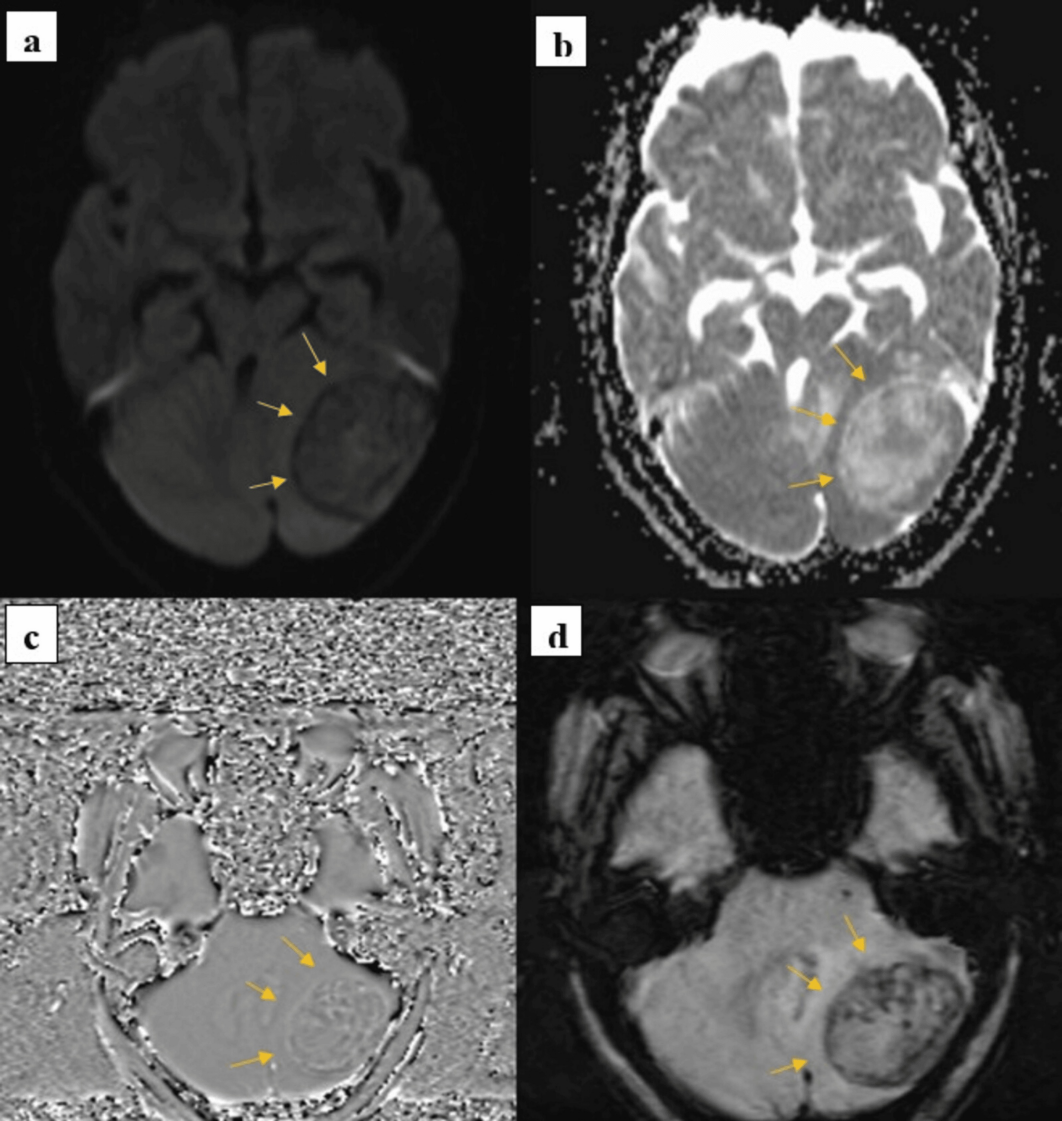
(a) Diffusion-weighted imaging (DWI) and (b) apparent diffusion coefficient (ADC): The lesion shows no evidence of restricted diffusion on DWI (yellow arrows). (c,d) A few blooming foci are noted on susceptibility-weighted imaging (SWI) within the lesion (yellow arrow), suggestive of calcifications.
